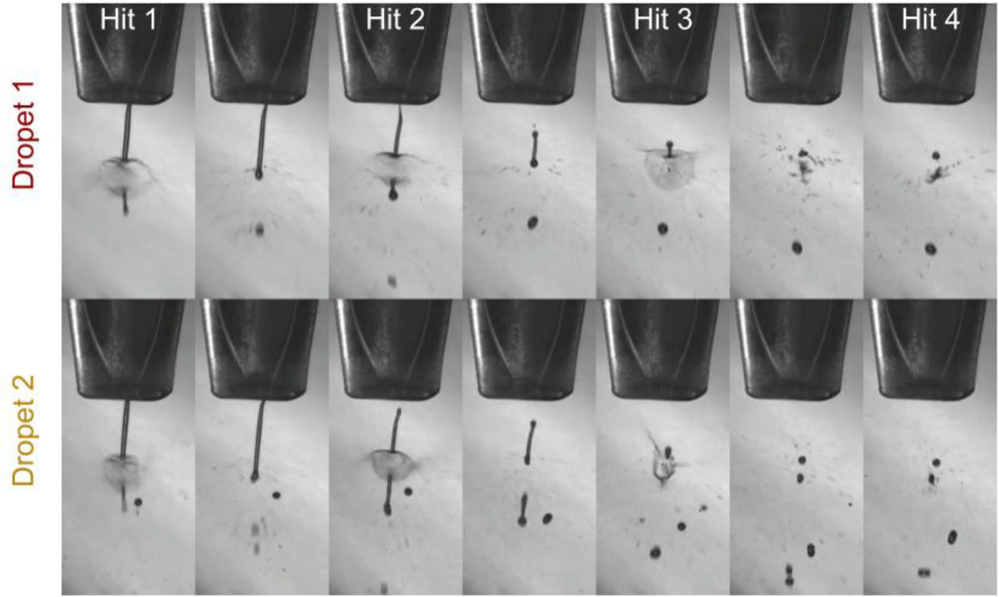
Droplet-on-demand injection scheme. Top: Images from the first Knackwurst (elongated droplet) ejection sequence, wherein the Knackwurst undergoes four successive hits (labelled hit 1 to 4), each marked by a notable explosion. Each frame is shown at intervals of 10.5 *μ*s. Bottom: A similar sequence for the second Knackwurst, in total four Knackwursts were ejected, each experiencing four hits. Consequently, 16 X-ray pulses were delivered over the macro-pulse at a frequency of 47 kHz. Note images at higher flux for droplet alignment and only display first two out of four Knackwursts.
